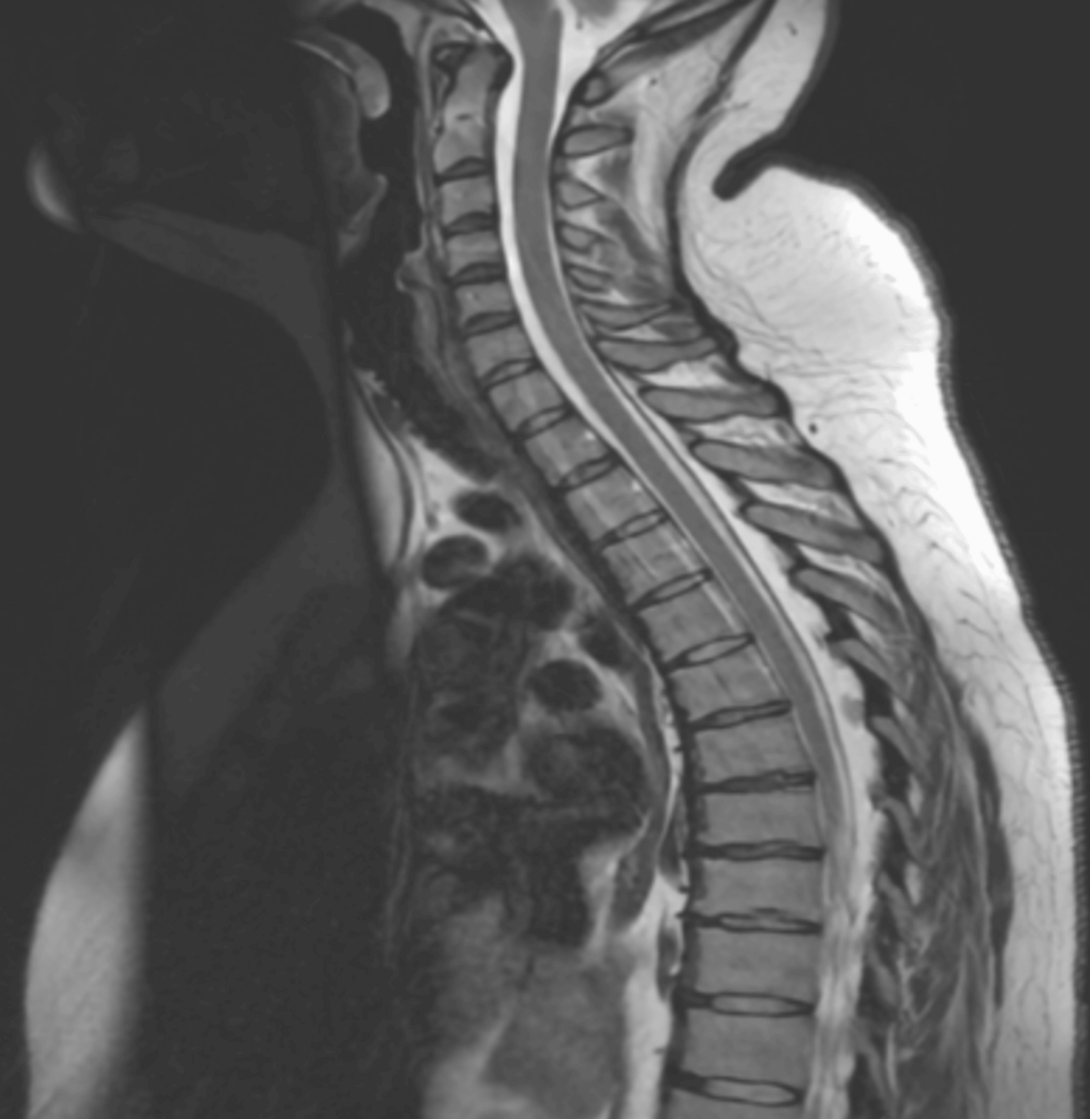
MRI Cervical and Thoracic Spines. Reported as normal.
